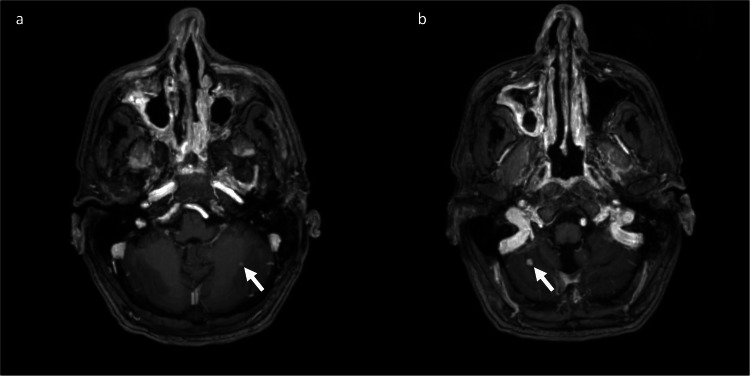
Postoperative cranial MRI with contrast medium (a) Axial T1-weighted MRI shows a contrast-enhancing mass in the left cerebellar hemisphere and (b) an axial T1-weighted MRI showing a contrast-enhancing mass in the right cerebellar hemisphere. Both masses are identical to the preoperative findings.
